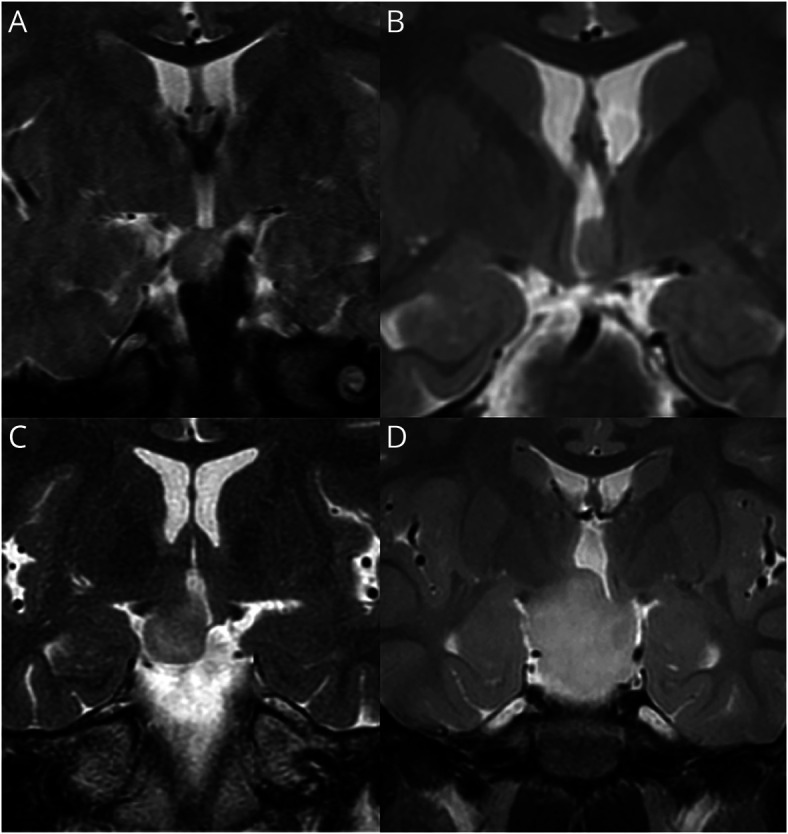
MRI Examples of Delalande Classification of Hypothalamic Hamartoma (HH) All panels show coronal T2-weighted MRI. HH lesion type is designated according to the proposed classification system of Delalande and Fohlen.^44^ (A) Type I HH: 2-year-old boy with mild developmental delay but otherwise asymptomatic. Note attachment of the HH lesion entirely below the floor of the third ventricle. (B) Type II HH: 5-year-old boy with a history of gelastic seizures since 12 months of age. Note attachment to the left side of the hypothalamus, entirely above the floor of the third ventricle. (C) Type III HH: 31-year-old woman with refractory epilepsy, including gelastic seizures since infancy. Note attachment of the HH lesion to the right side of the hypothalamus both above and below the floor of the third ventricle. (D) Type IV HH: 3-year-old girl with severe developmental delay, history of precocious puberty, and multiple daily gelastic seizures. Type IV is designated by Delalande and Fohlen as a “giant” HH, but criteria to distinguish between types III and IV were not offered. We (Barrow Neurologic Institute) currently use a volume measurement over 8 cm^3^ to designate type IV, measuring the maximal length of the lesion in the 3 major axes, then utilizing the formula for determining the volume of an ellipsoid (courtesy of John F. Kerrigan, MD, Barrow Neurologic Institute).
